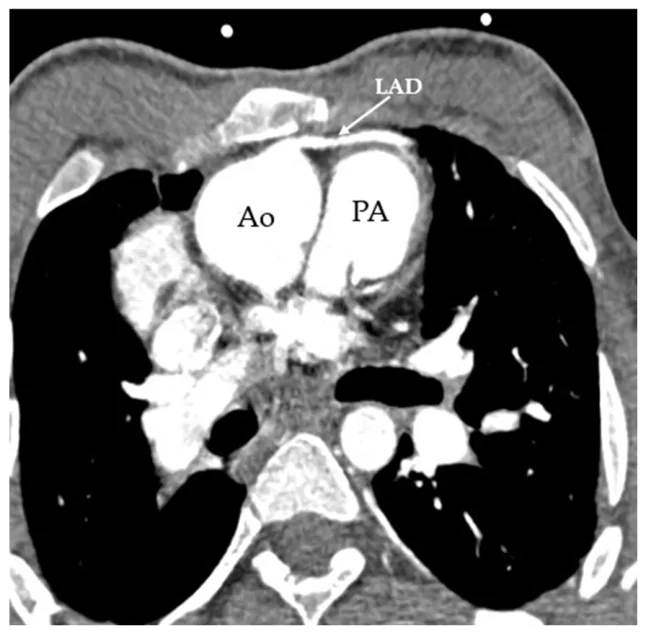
Computed tomography scan showing anomalous left anterior descending (LAD) coronary artery crossing the right ventricular outflow tract in an adult patient with tetralogy of Fallot; Ao: aorta; PA: pulmonary artery; LAD: left anterior descending coronary artery.
